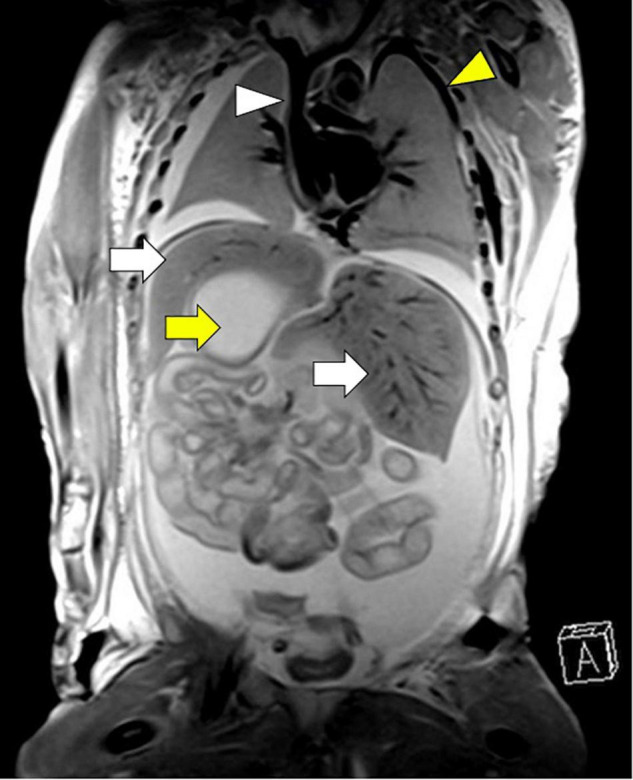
Concordant pattern of broncho-pulmonary branching confirmed by post-mortem MRI in the left atrial isomerism cohort. Arrows: white, bilateral liver; yellow, right-sided stomach. Arrowheads: white, right atrium with insertion of vena cava superior, but without vena cava inferior, yellow, vena azygos supplying blood of the lower body half. Gestational age at MRI is 23 weeks + 4 days.
